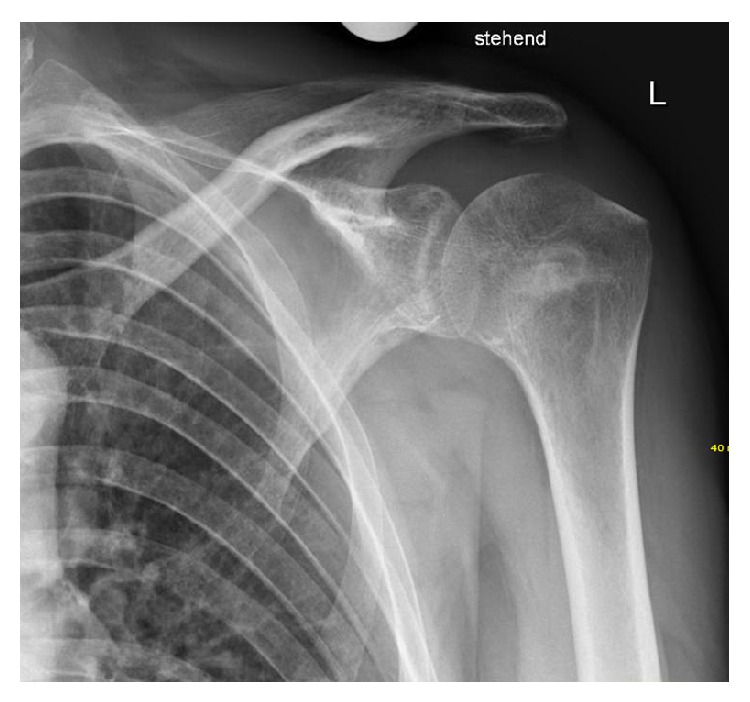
X-ray (a.p. view) at 6-month follow-up documenting the bony remodeling of the bone substitute.
